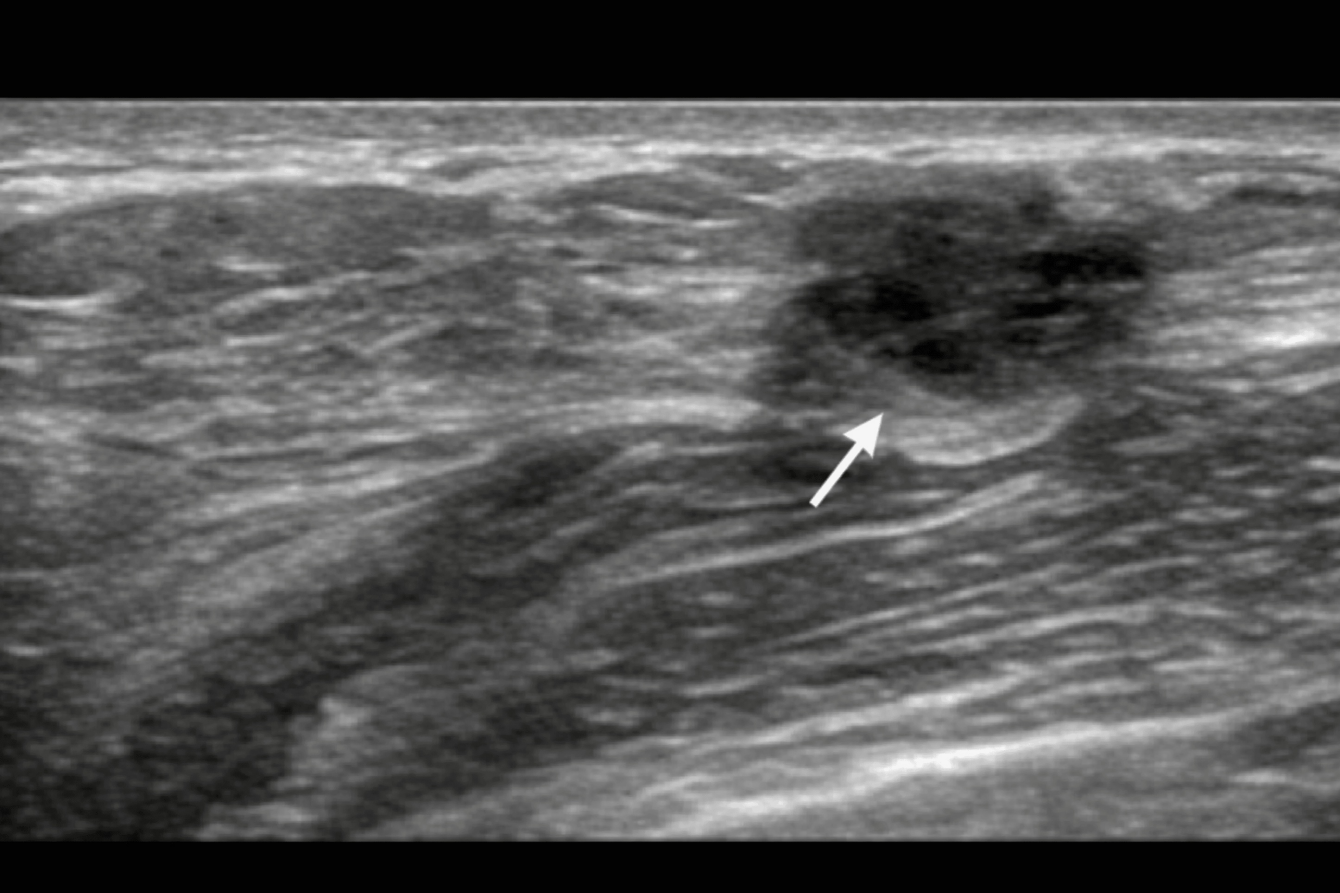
Targeted breast ultrasound confirming a corresponding irregular hypoechoic mass at the 2 o’clock position.
